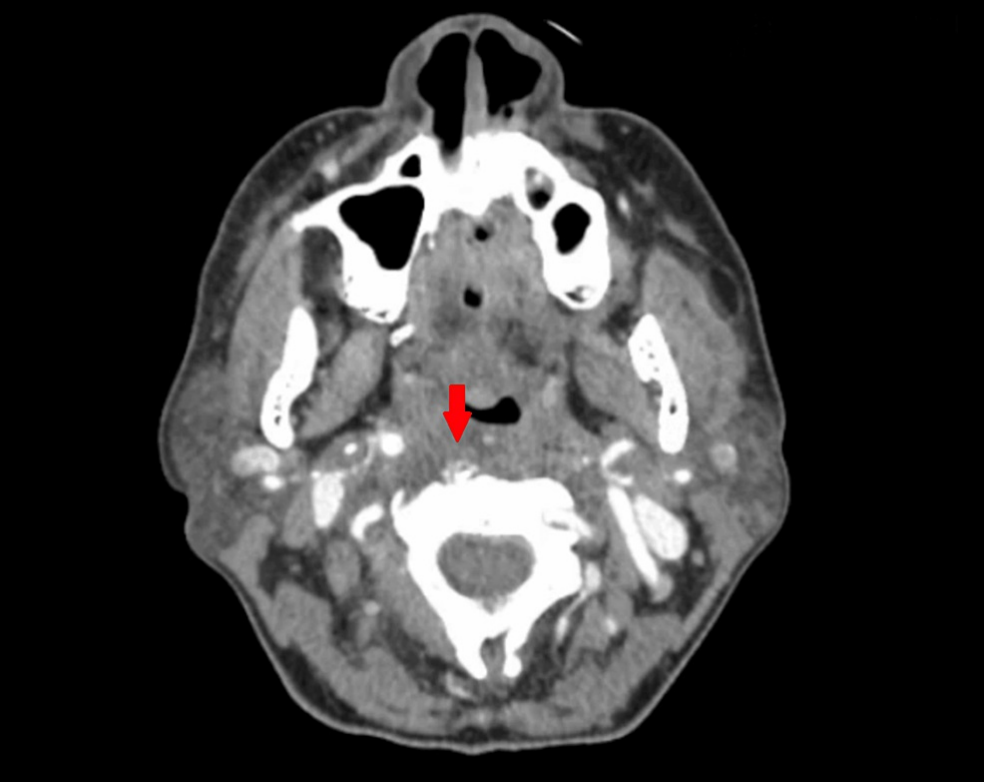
Contrast-enhanced computed tomography (CT) of the neck (axial view) Amorphous calcification of the superior fibers of the longus colli tendons (red arrow)
